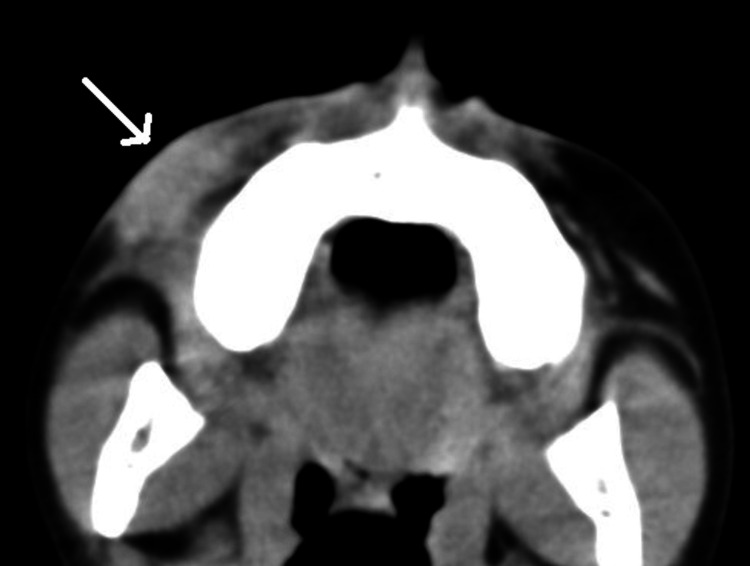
Enhanced CT scan of the face showing an enhancing subcutaneous soft tissue lesion of the right cheek (arrow)
